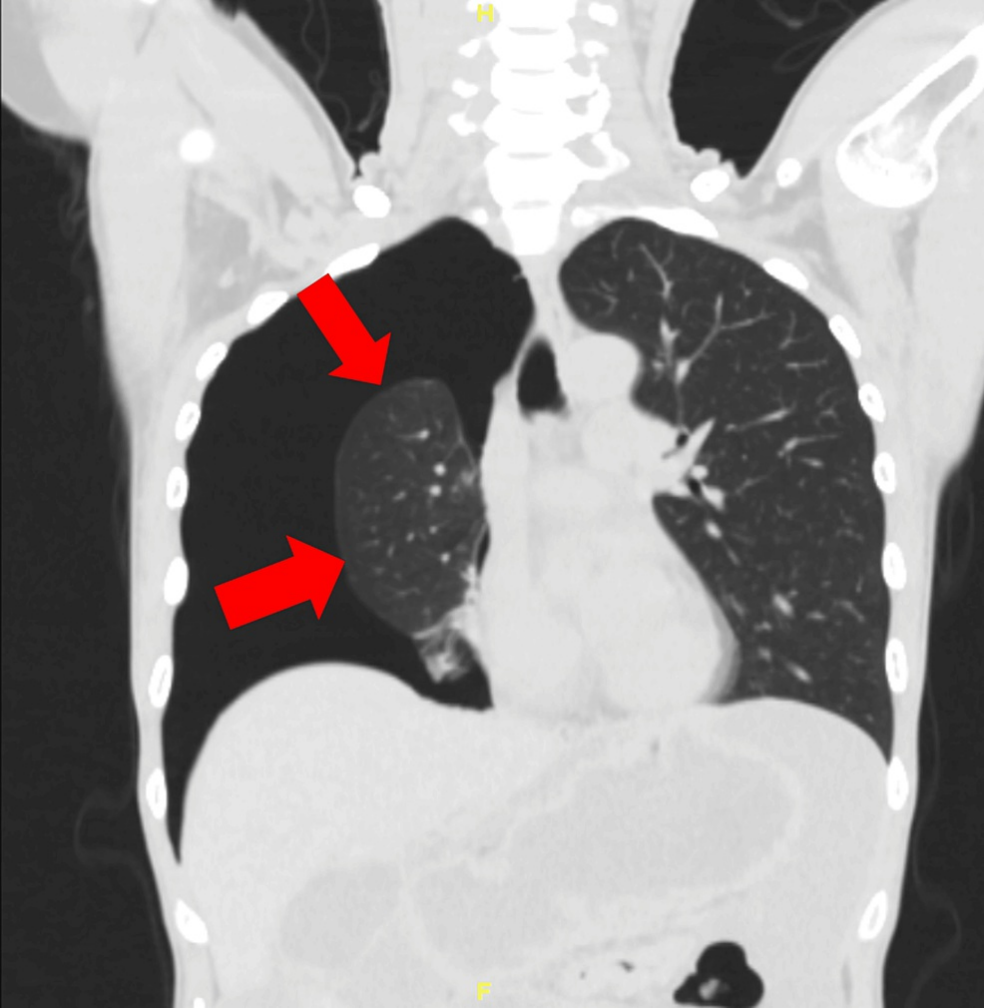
CT chest showing collapsed right lung with arrows indicating the visible pleural edge line
